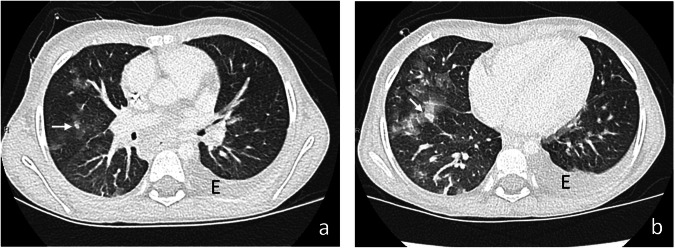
Proven invasive pulmonary aspergillosis in a 4-year-old girl with acute myeloblastic leukemia (AML) under chemotherapy. Chest CE-CT scan performed because of increasing cough and fever evidenced multiple patchy areas of ground-glass attenuation and pulmonary nodules (white arrows a and b) surrounded by ground-glass opacity (“halo sign”). Note also moderate left pleural effusion (E). Diagnosis was confirmed by bronchoalveolar lavage
